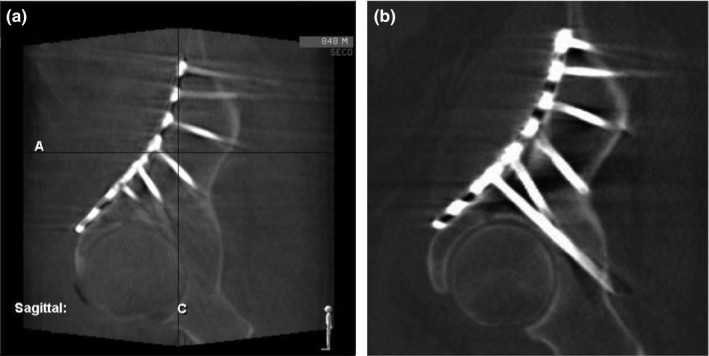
Postsurgical sagittal reconstruction of patient hardware from the (a) Ziehm c‐arm and (b) multislice CT scanner.
